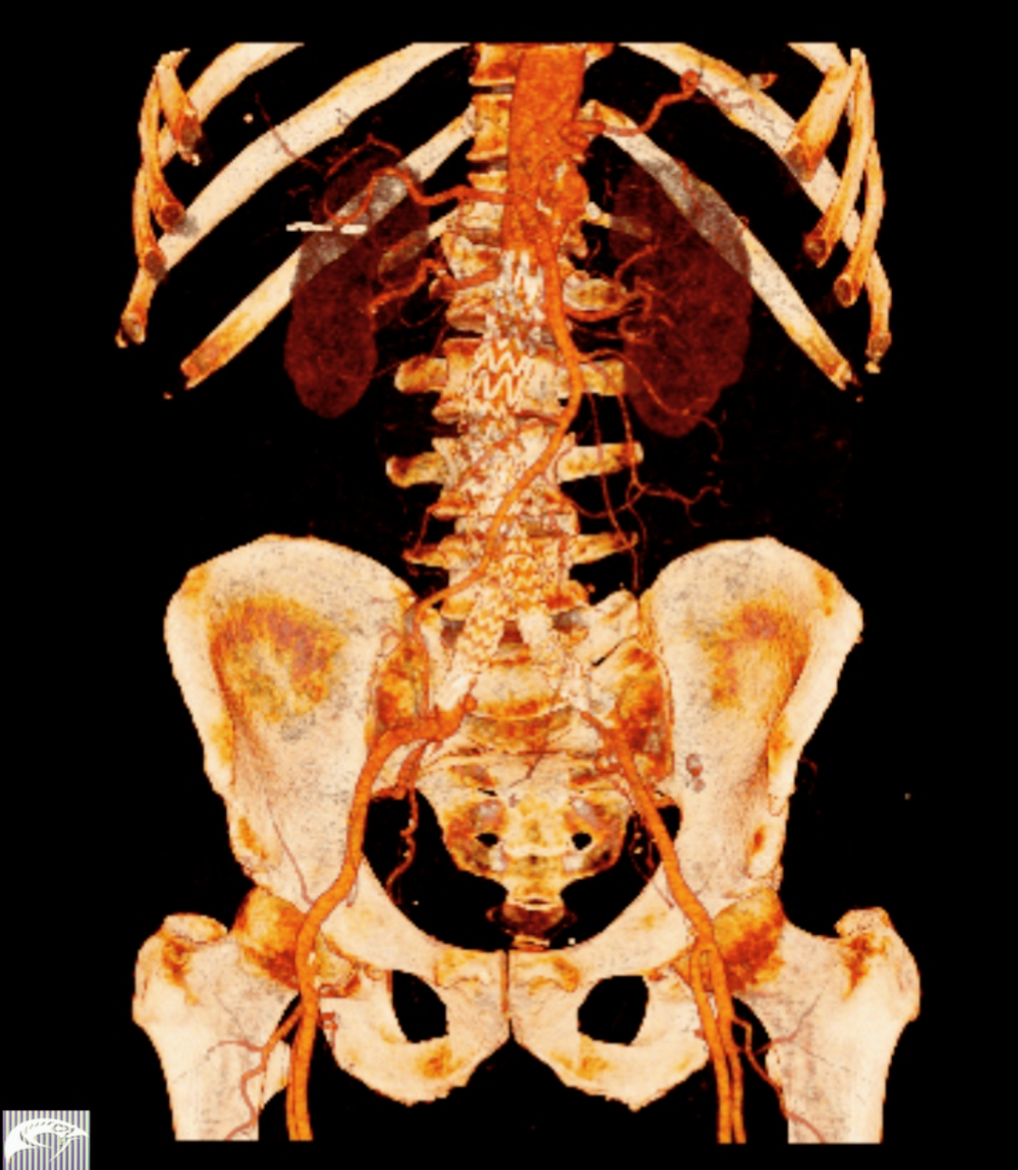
Postoperative computed tomography angiography showing the bifurcated abdominal aortic stent-graft in place, without evidence of endoleak
